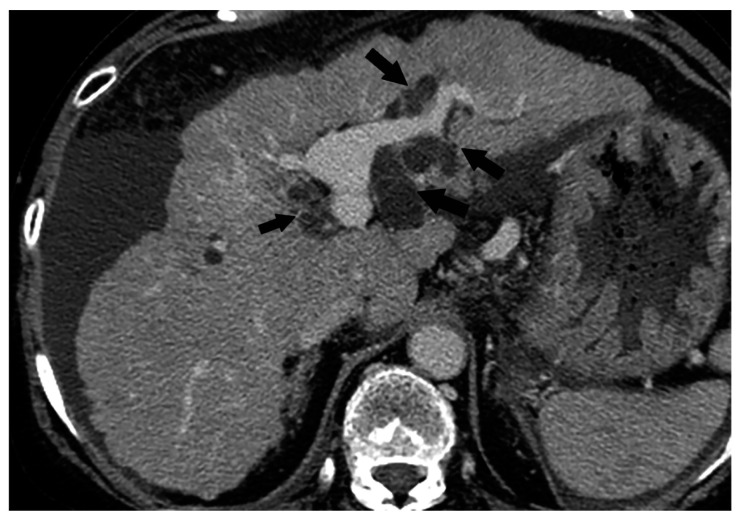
Peribiliary cyst observed in a 56-year-old man with cirrhosis. In the portal venous phase on contrast-enhanced CT, dilated multiple hypodense cystic structures (arrows) are observed along the left main portal vein and its branch in the hepatic hilar area. These structures are peribiliary cysts, showing cystic dilatation of the peribiliary glands around the large bile duct. Although this hypodense structure looks like intrahepatic bile duct dilatation, it is located bilaterally along the portal vein, unlike dilated bile duct (arrows).
